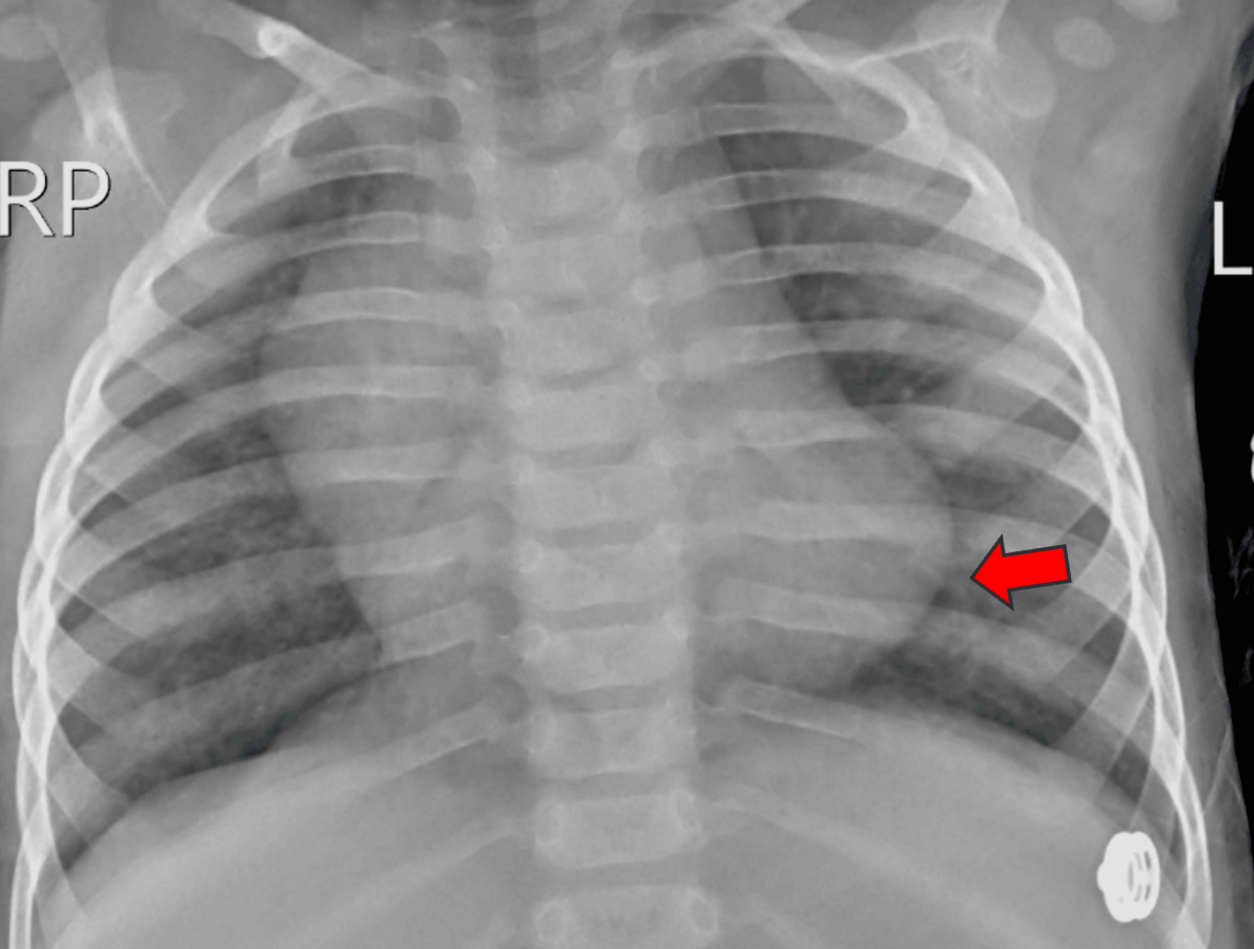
Chest X-ray showing cardiomegaly (red arrow)
